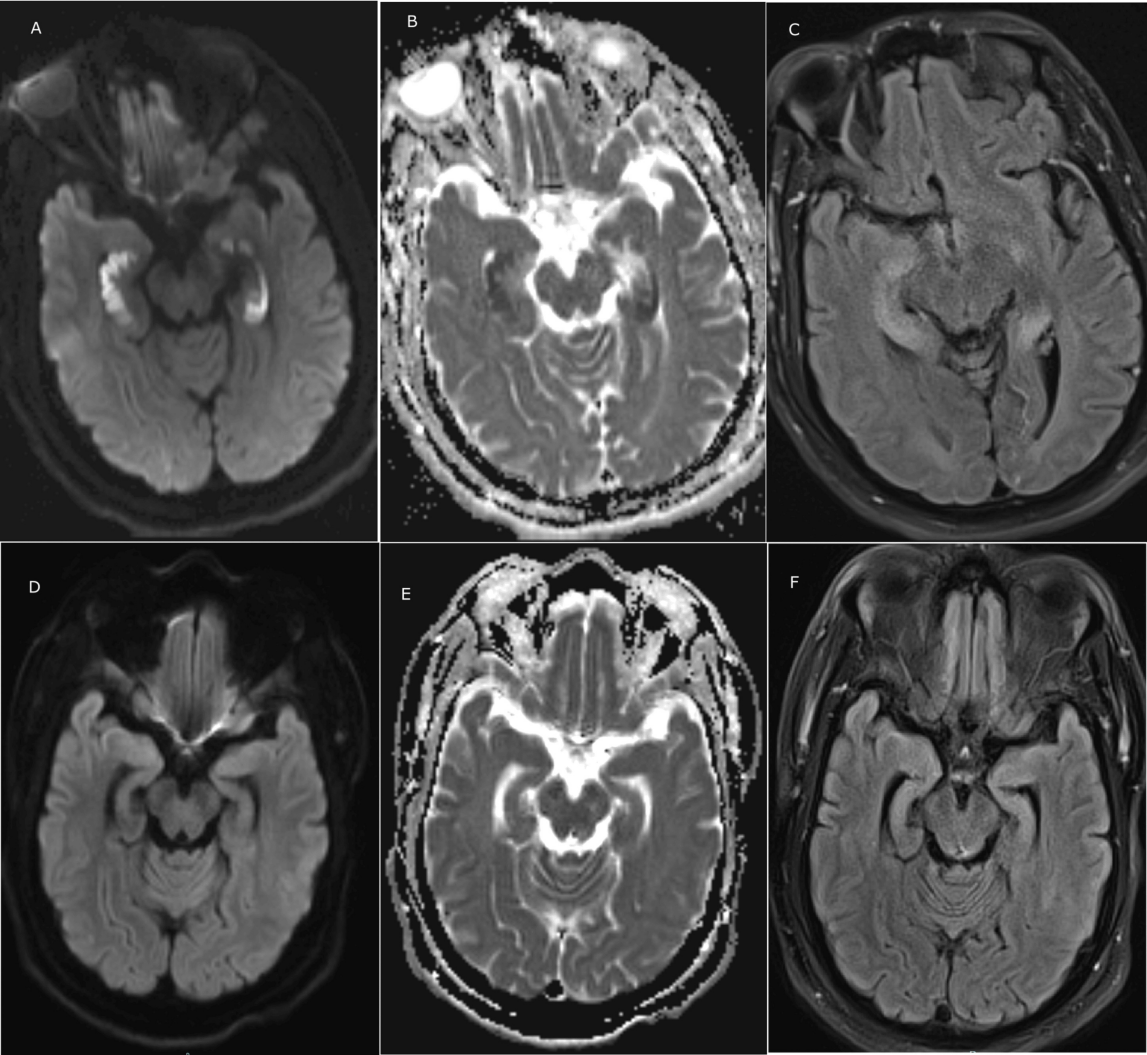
MRI images of the bilateral hippocampi at presentation and at the three-month follow-up ADC/DWI images show diffusion restriction and T2 FLAIR shows edema of the bilateral hippocampi at presentation and at the three-month follow-up A. Diffusion-weighted imaging (DWI) image at presentation; B. Apparent diffusion coefficient (ADC) image at presentation; C. T2 fluid-attenuated inversion recovery (FLAIR) image at presentation; D. DWI image at three months; E. ADC image at three months; F. T2 FLAIR image at three months.
